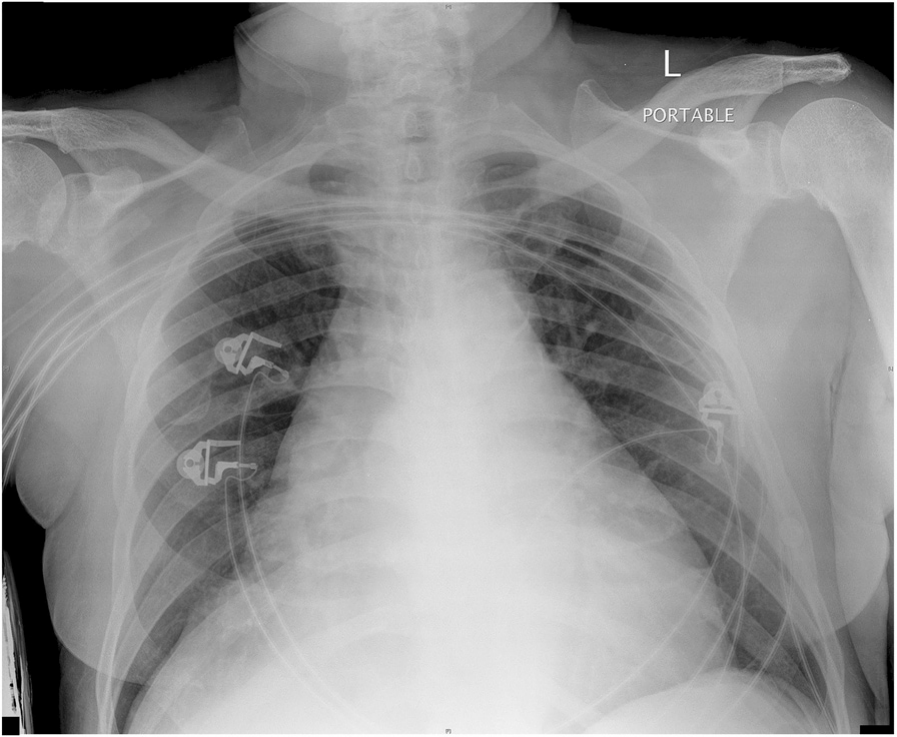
Preoperative antero-posterior chest radiograph. Antero-posterior chest radiograph shows widened mediastinum and parenchymal haziness at the bases of both lungs.
